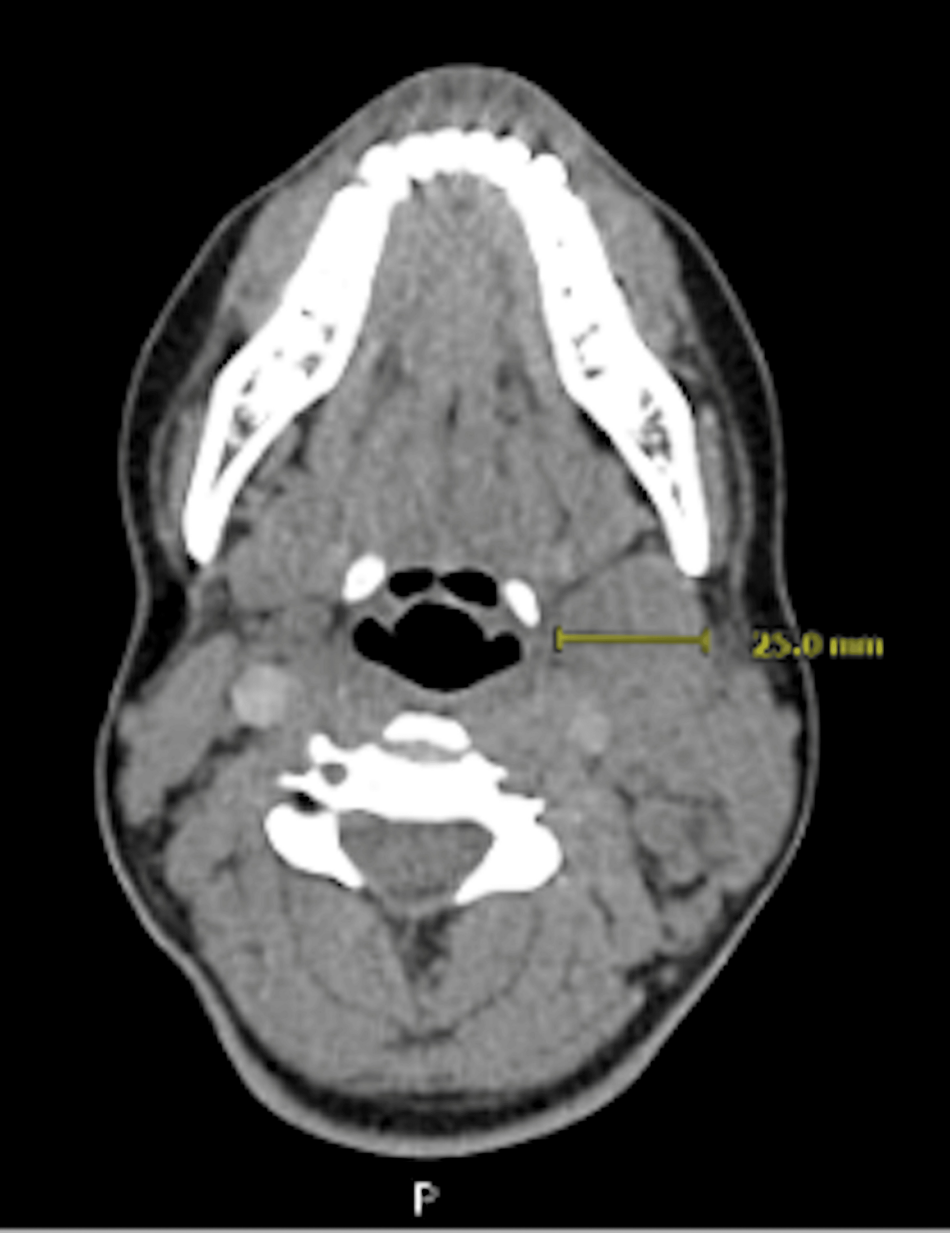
Representative CT image showing swelling on the left side of the patient’s neck Axial CT image of the neck with contrast showing large level II matted left cervical adenopathy, measuring 2.5 cm in greatest dimension. CT, computed tomography
